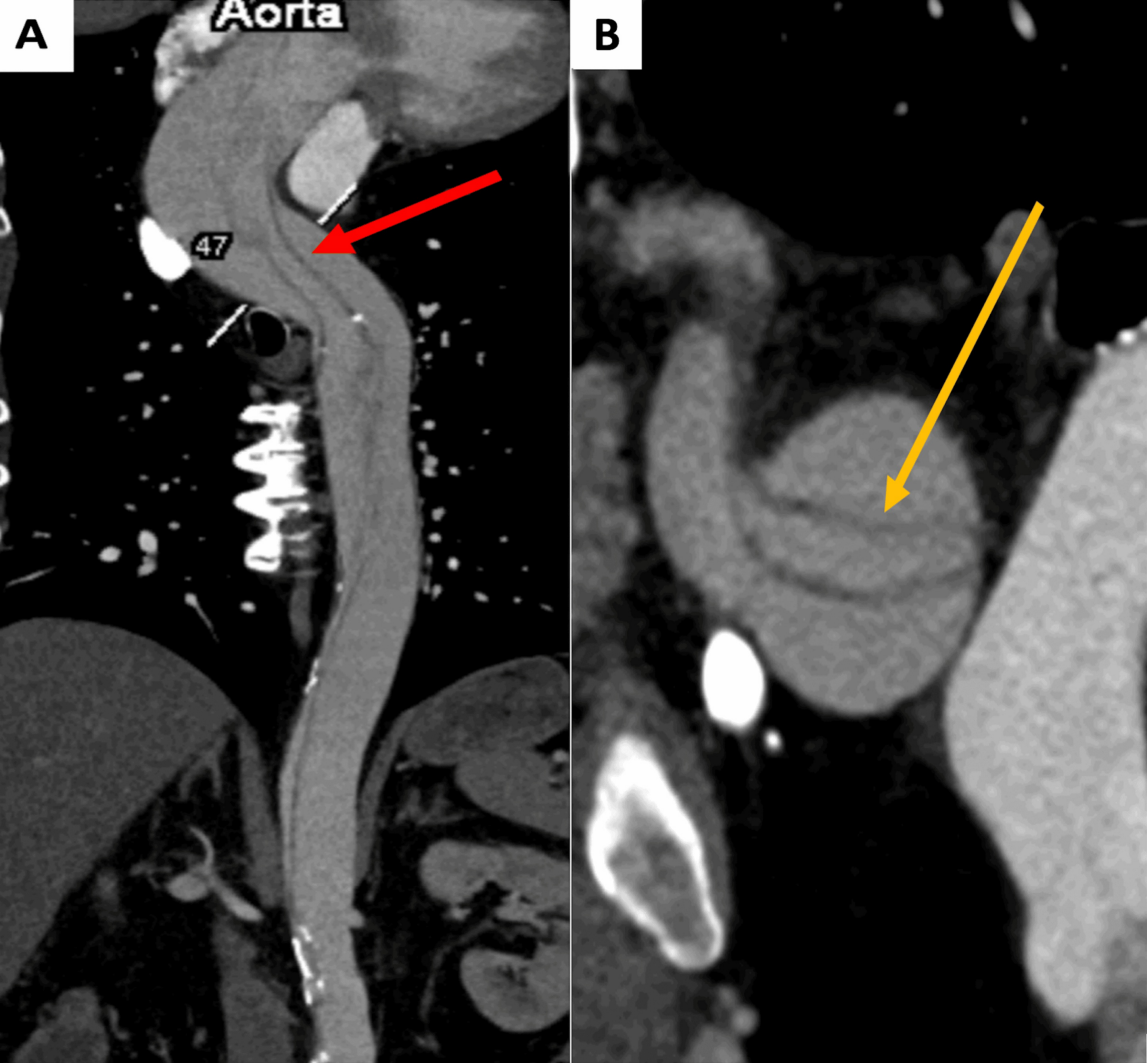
A-B: CT angiography reconstructions revealing an extensive aortic dissection involving the ascending, arch, descending thoracic, and abdominal aorta (A) Longitudinal extent of the dissection (marked with the red arrow). (B) Cross-sectional view demonstrating the separation between the true and false lumens (marked with the yellow arrow). CT: computed tomography
